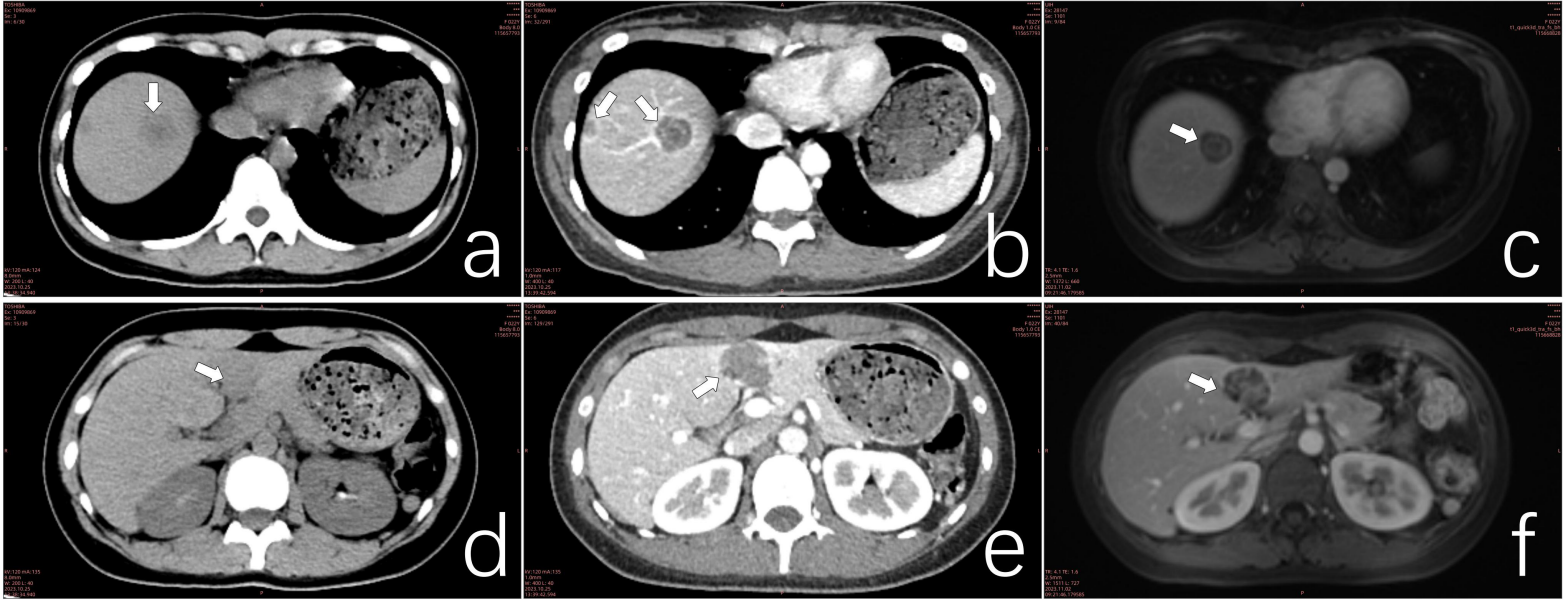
(a) CT scan of a lesion in hepatic segment VIII. (b) Contrast-enhanced CT scan of the segment VIII lesion exhibiting the target sign. (c) MRI of segment VIII lesions demonstrating the double ring sign. (d) CT scan of confluent lesions in hepatic segments III and IV. (e) Contrast-enhanced CT scan of confluent lesions in segments III and IV showing mild enhancement. (f) MRI of confluent lesions in segments III and IV, revealing heterogeneous internal signals.
